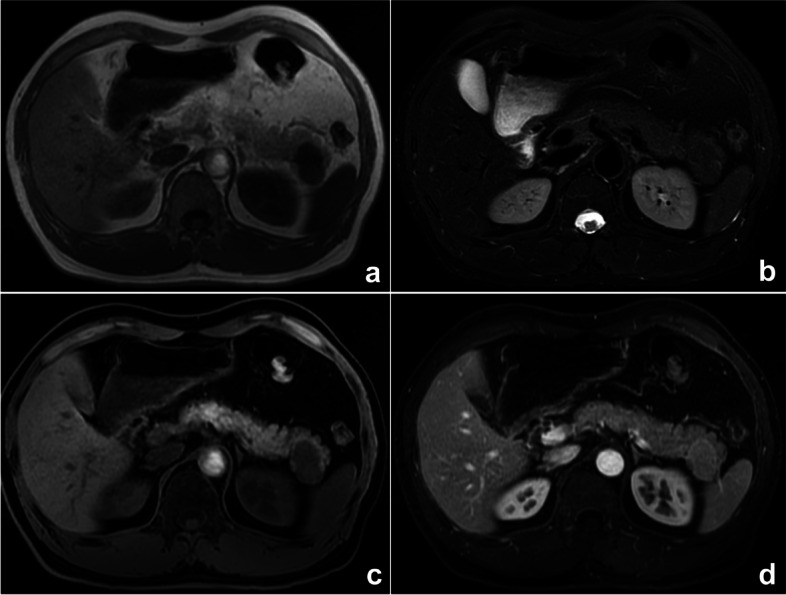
A space-occupying lesion of the pancreatic tail in a 52-year-old man. Axial MRI T_1_WI (a), T_2_WI/FS (b), T_1_WI/FS (c), and enhanced (d) sequences all showed that the lesion’s signal was similar to that of the spleen, but our radiologist’s diagnosis was pancreatic neuroendocrine tumor, which was relatively common, rather than a rarely occurring ectopic spleen. Finally, the surgical pathology confirmed an ectopic spleen in the pancreas. This thinking bias is classified as zebra retreat bias
